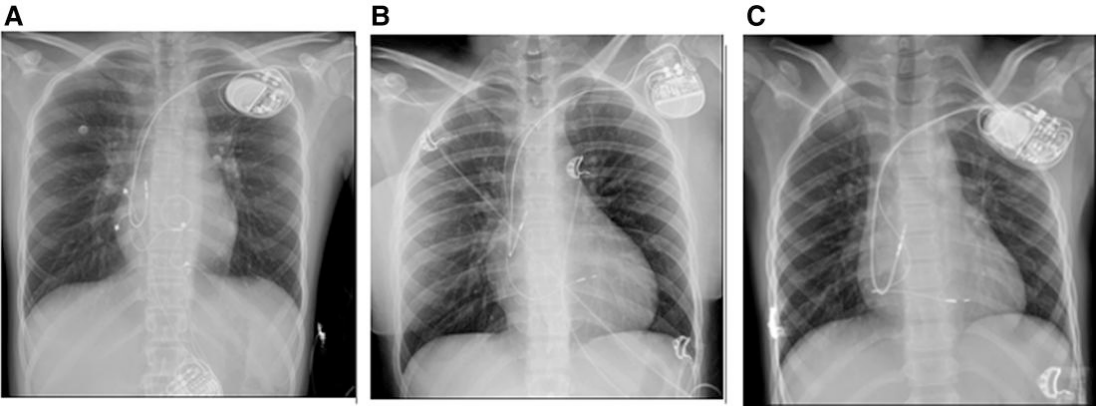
X-ray image 1 day after implantation: Case 1 (*A*), Case 2 (*B*), and Case 3 (*C*).
